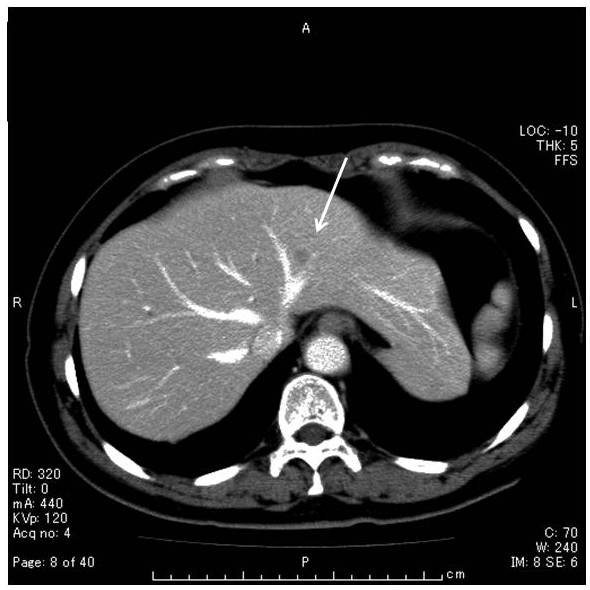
Unenhanced CT scan showed low density area of 1 cm in diameter in the segment 3 of the liver (arrow). Contrast-enhanced CT scan during arterial phase showed minimally peripheral ring enhancement. No lymphadenopathy or hepatosplenomegaly was observed.
